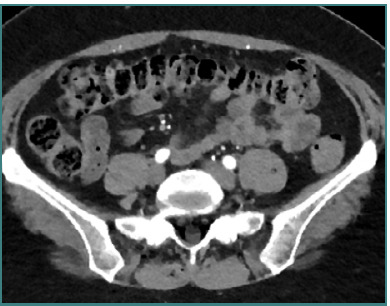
Axial view of L5-S1 level on CTA
